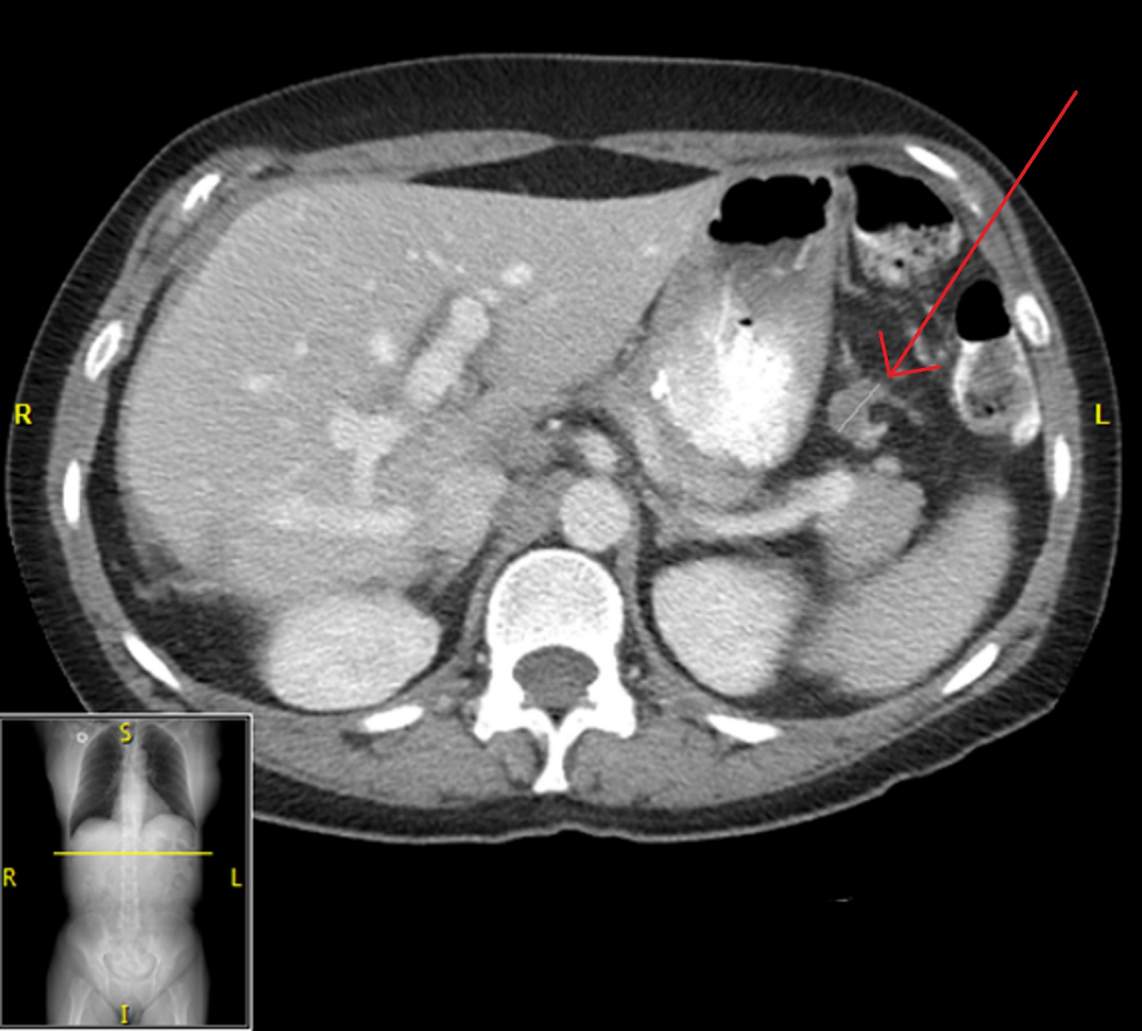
Abdominal CT of Patient 2 prior to cycle three of evofosfamide, showing a disappearance of peritoneal implants along with a reduction in size of target lesion to 1.8 cm in maximal dimension; CA-125 1602 U/ml
